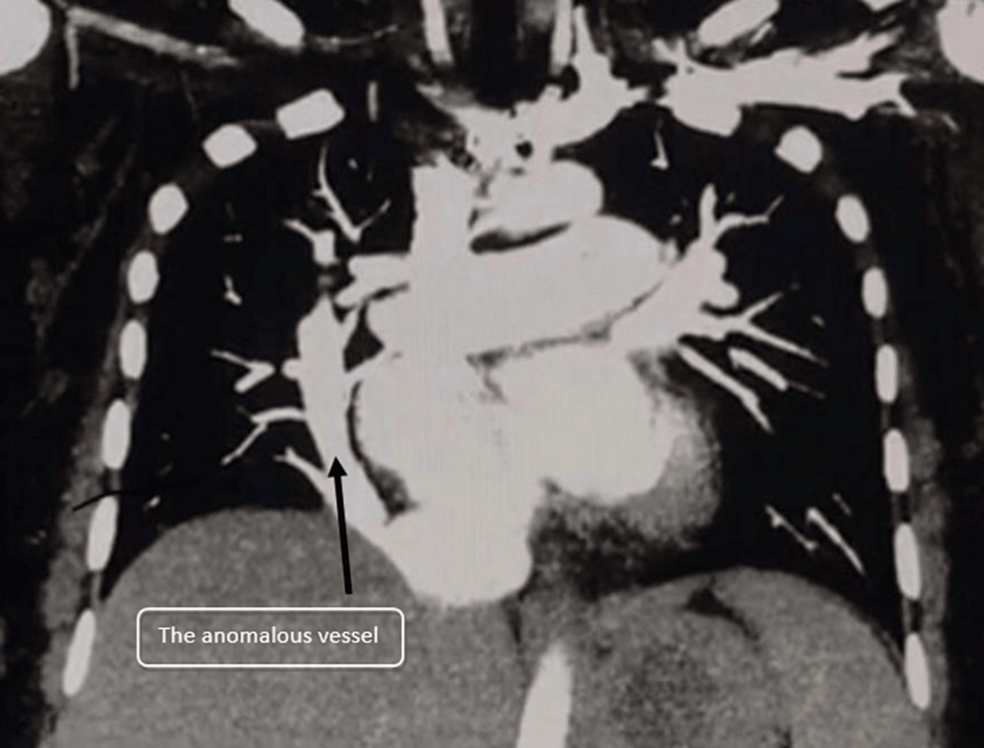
A CTPA with a peculiar vessel originating from the medial aspect of the right hemidiaphragm. CTPA: computed tomography pulmonary angiography
